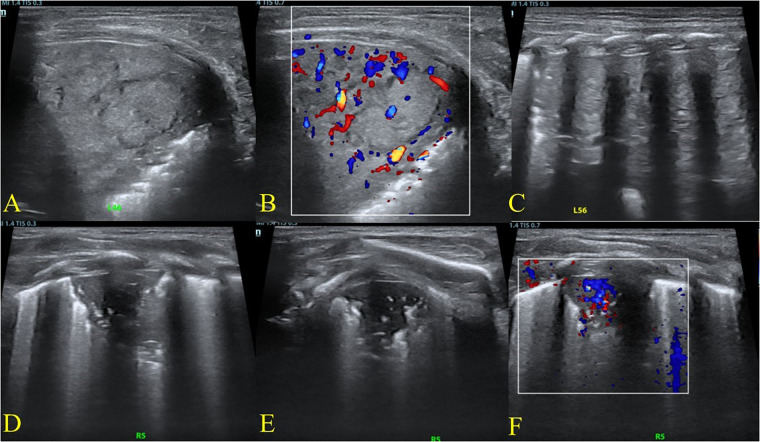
Serial lung ultrasound findings on hospital day 2 under conventional mechanical ventilation. Ultrasound on hospital day 2, under conventional mechanical ventilation, showed persistent heterogeneous consolidation of the left lung with detectable intralesional blood flow (A–C). The right lung consolidation had markedly decreased, limited to the posterior upper region, with visible air bronchograms and blood flow signals (D–F).
